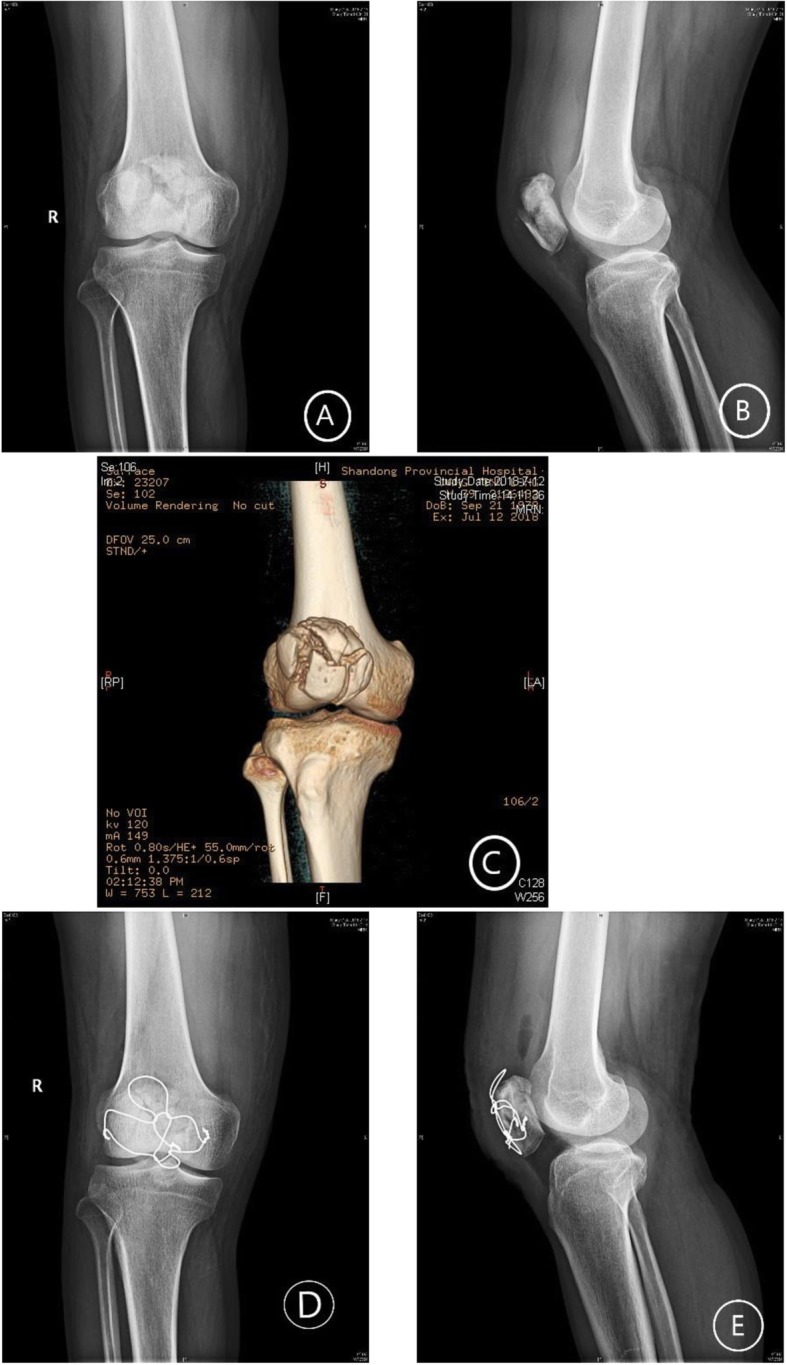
A 38-year-old male with severely comminuted patellar fracture. a, b X-ray before surgery. c CT before surgery. d, e Post-fixation with modified cerclage wiring
